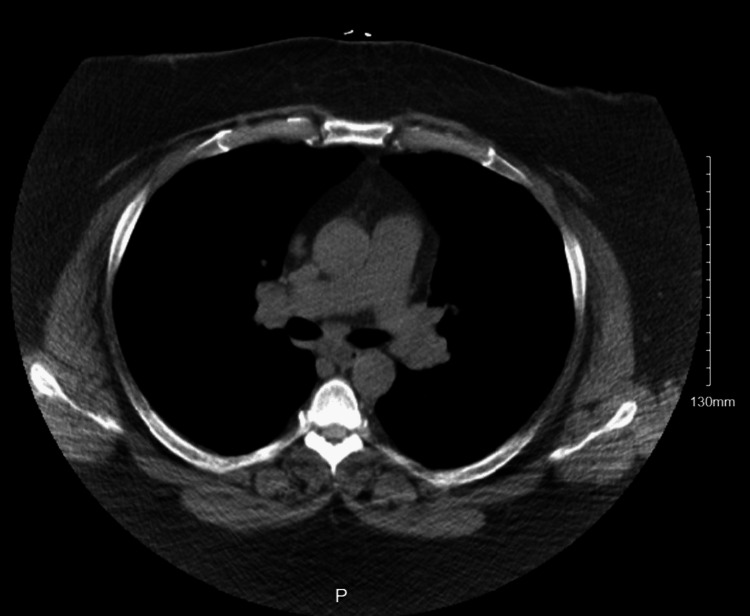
CT chest showing bilateral hilar adenopathy
